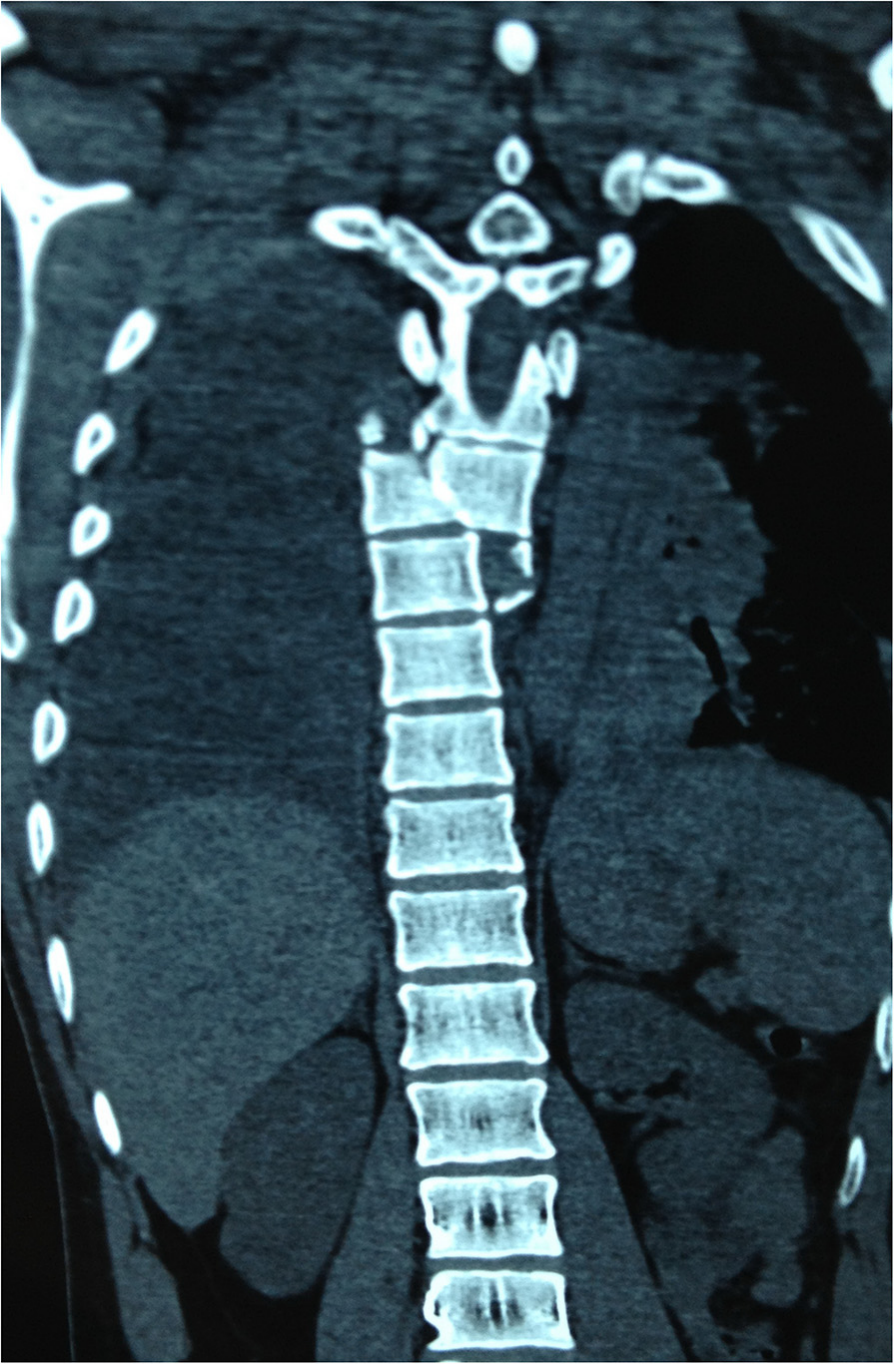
Three-dimensional reconstruction of computed tomography showed spinal fracture-dislocation of T6 to T7.
